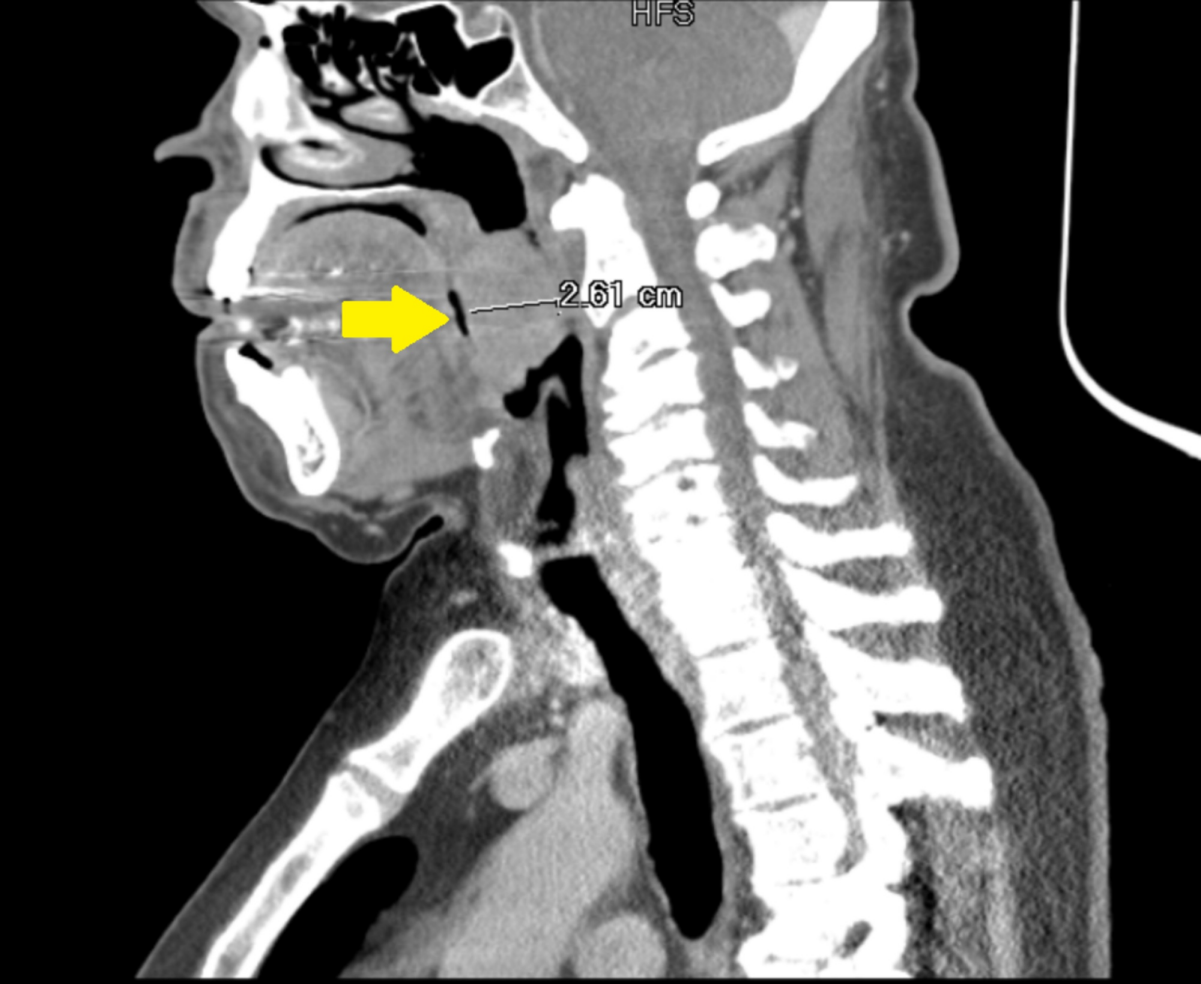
Computed tomography (CT) of the soft tissue of the neck A 2.6-cm mass can be seen obstructing the oropharyngeal airway (yellow arrow).
